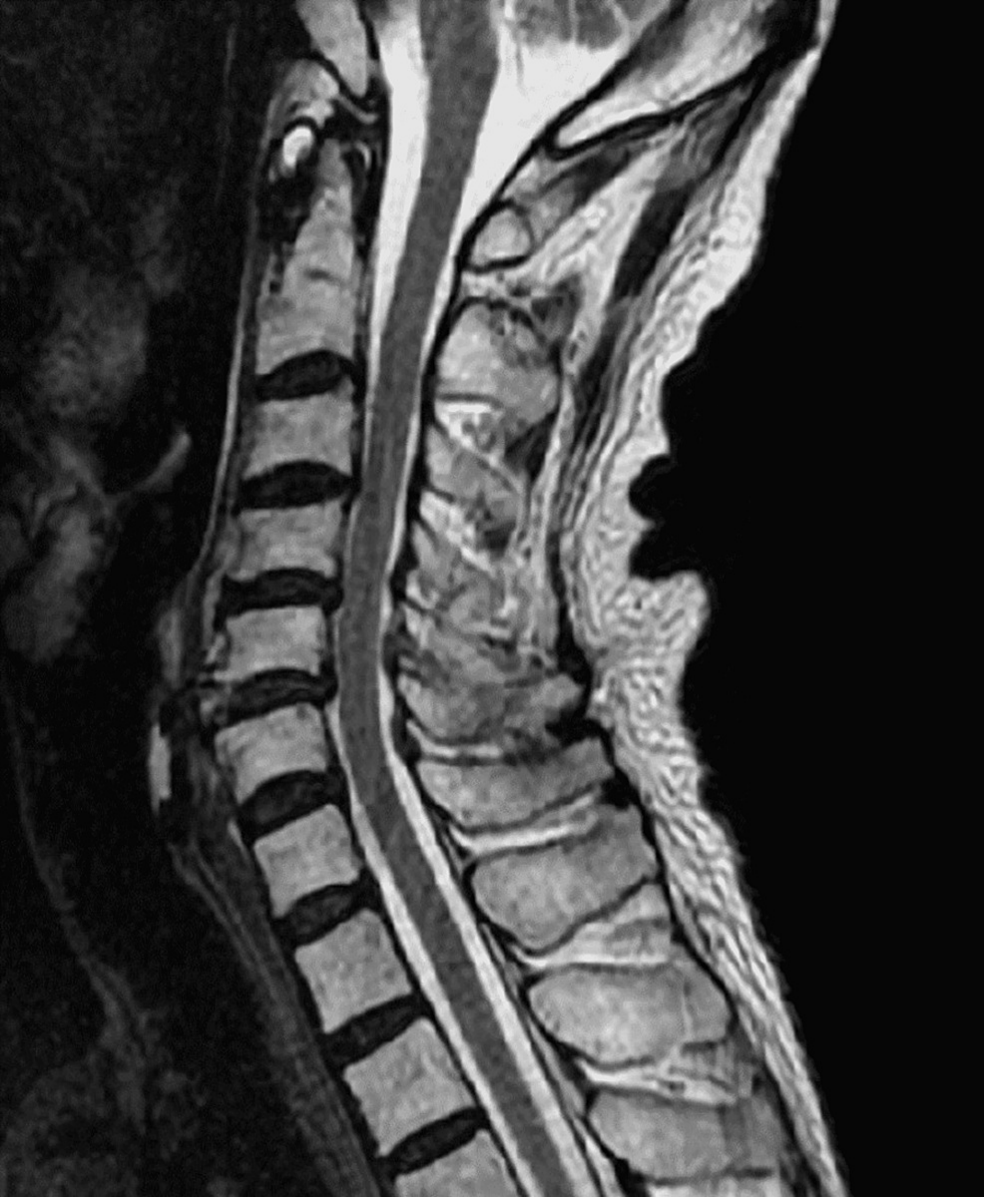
T2-weighted MRI of the neck does not show demyelination.
